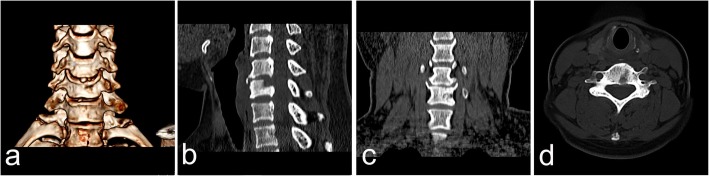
CT images obtained 3 months after the operation, including the axial plane view (a), coronal reconstruction view (b), sagittal reconstruction view (c), and three-dimensional view (d), showed that the bone defect had almost completely disappeared. Neither disc space narrowing nor instability were observed
